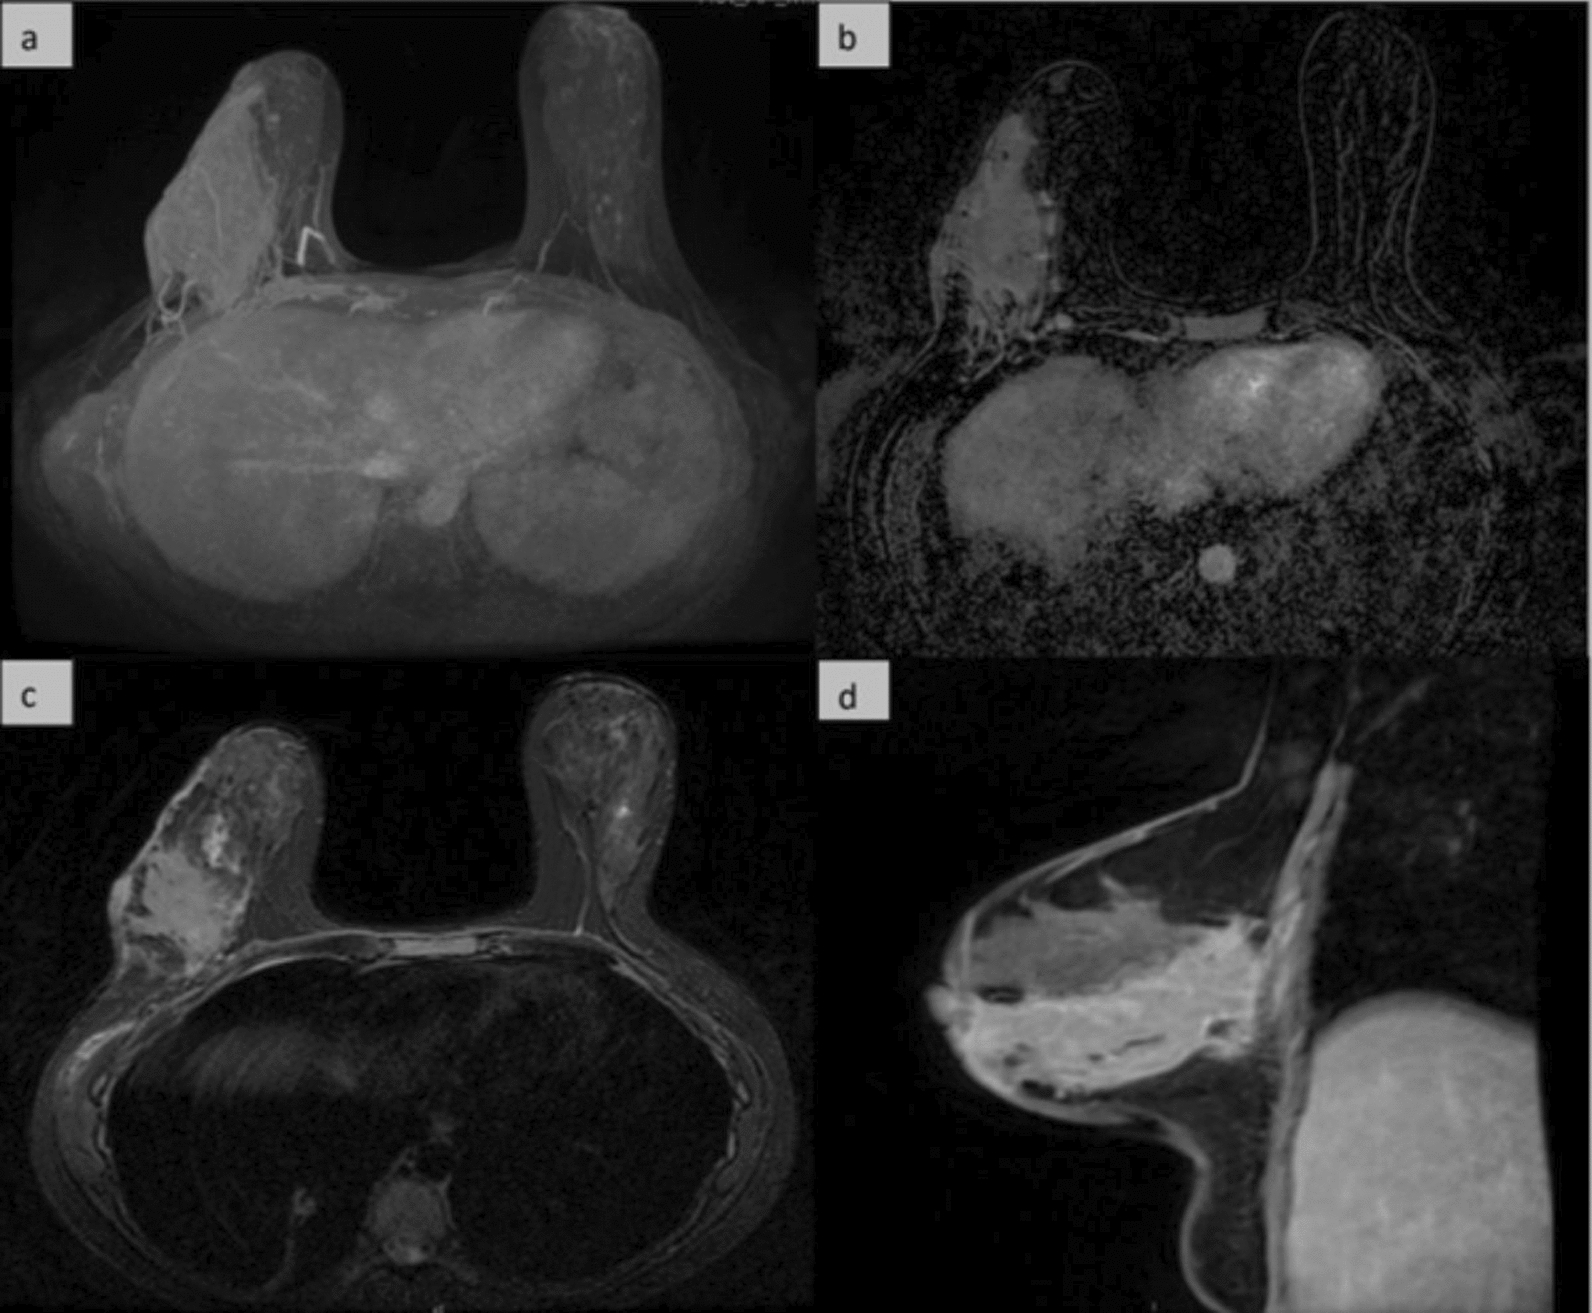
Magnetic resonance imaging at diagnosis before starting therapy: a MIP on axial plane, b post contrast subtracted T1-weighted image with fat saturation and c and no-subtracted on axial plane and d sagittal plane show an extensive soft tissue enhancing area involving the outer quadrants of the right breast, from the nipple to the deep planes posteriorly, with skin thickening. *MIP* Maximum intensity projection
